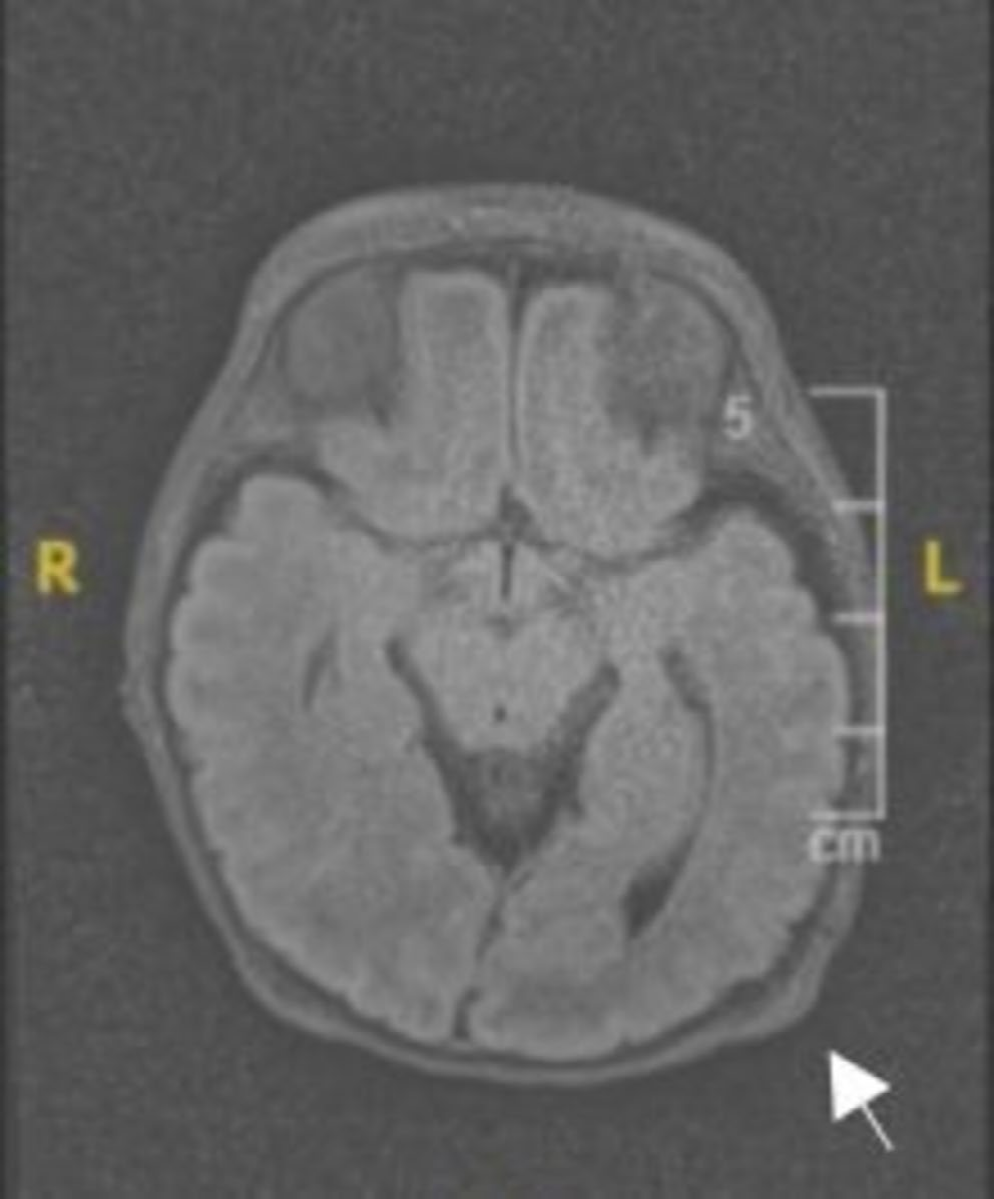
Axial T2‐weighted MRI of the occipital region in a preterm neonate. White arrow indicate well‐defined extracranial soft tissue nodules along the occipital scalp. There is no intracranial extension.
